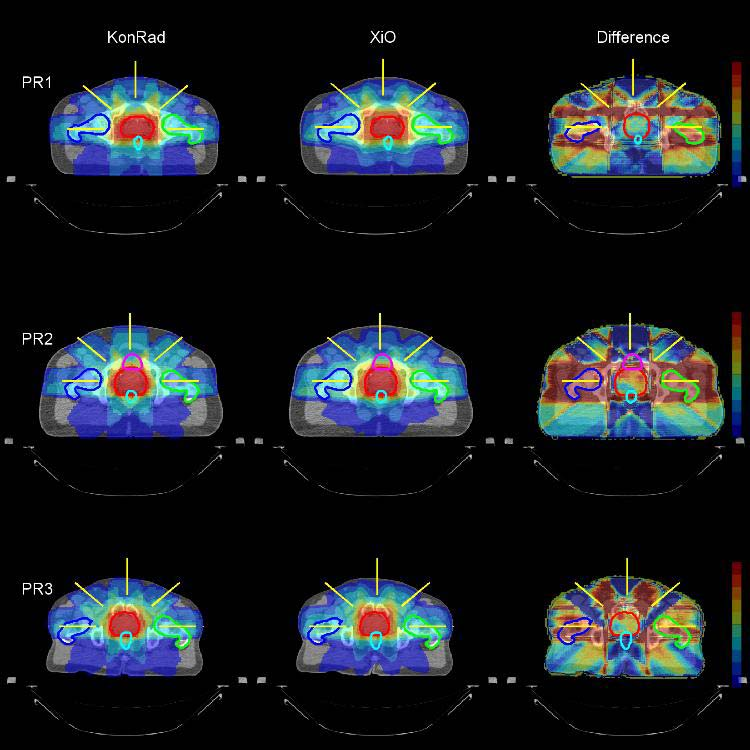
Beam arrangement and dose distribution of the prostate cases PR1, PR2 and PR3 from the KonRad (left column) and XiO (middle column) systems as well as the dose difference between the two plans (right column). The beam directions are indicated as yellow lines on a transverse CT slice through the isocenter. The contours of the PTVs are shown in red, the left and right femoral heads in green and blue, and the rectum in cyan. The dose distribution for the two plans is shown as a color wash overlay. The right column shows the difference of the dose distributions. The color wash for the dose difference ranges from +5 Gy in dark red to −5 Gy in dark blue, where positive numbers indicate a higher dose value in the XiO plan.
